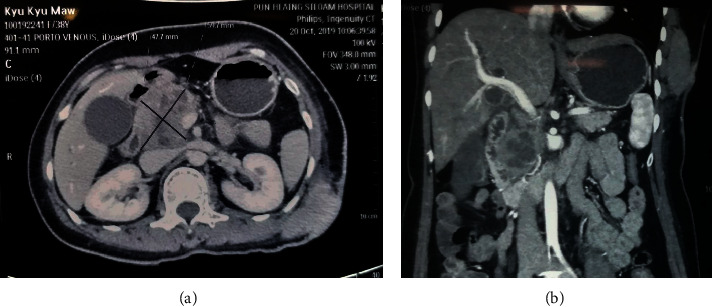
(a), (b) CT revealed malignant cystic pancreatic tumor occupying head and body with invasion to lower end of CBD and periampullary region causing biliary obstruction.
